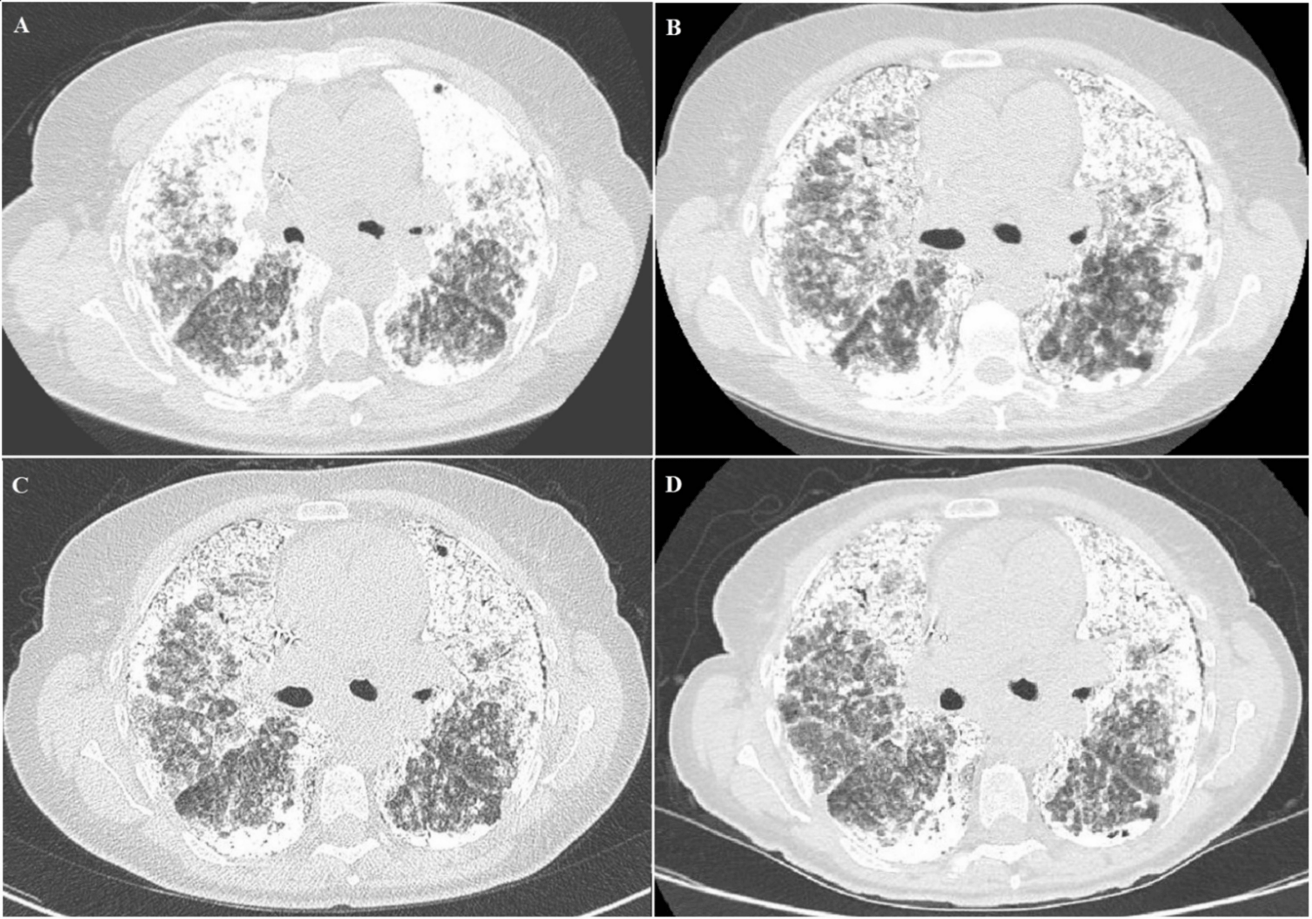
Radiological progression HRCTs: high-resolution CT scans High-resolution CT scans were performed in our institution during the long-term follow-up. HRCTs were performed at 22 years (A), 27 years (B), 28 years (C), and 31 years (D) since PAM diagnosis. No clear radiological progression was demonstrated during the last nine years of follow-up
